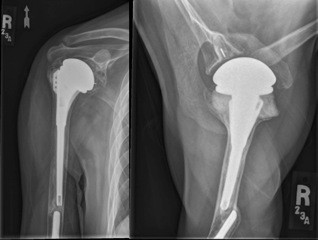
Anteroposterior and axillary radiographs when the patient first presented with some pain in the humerus.
